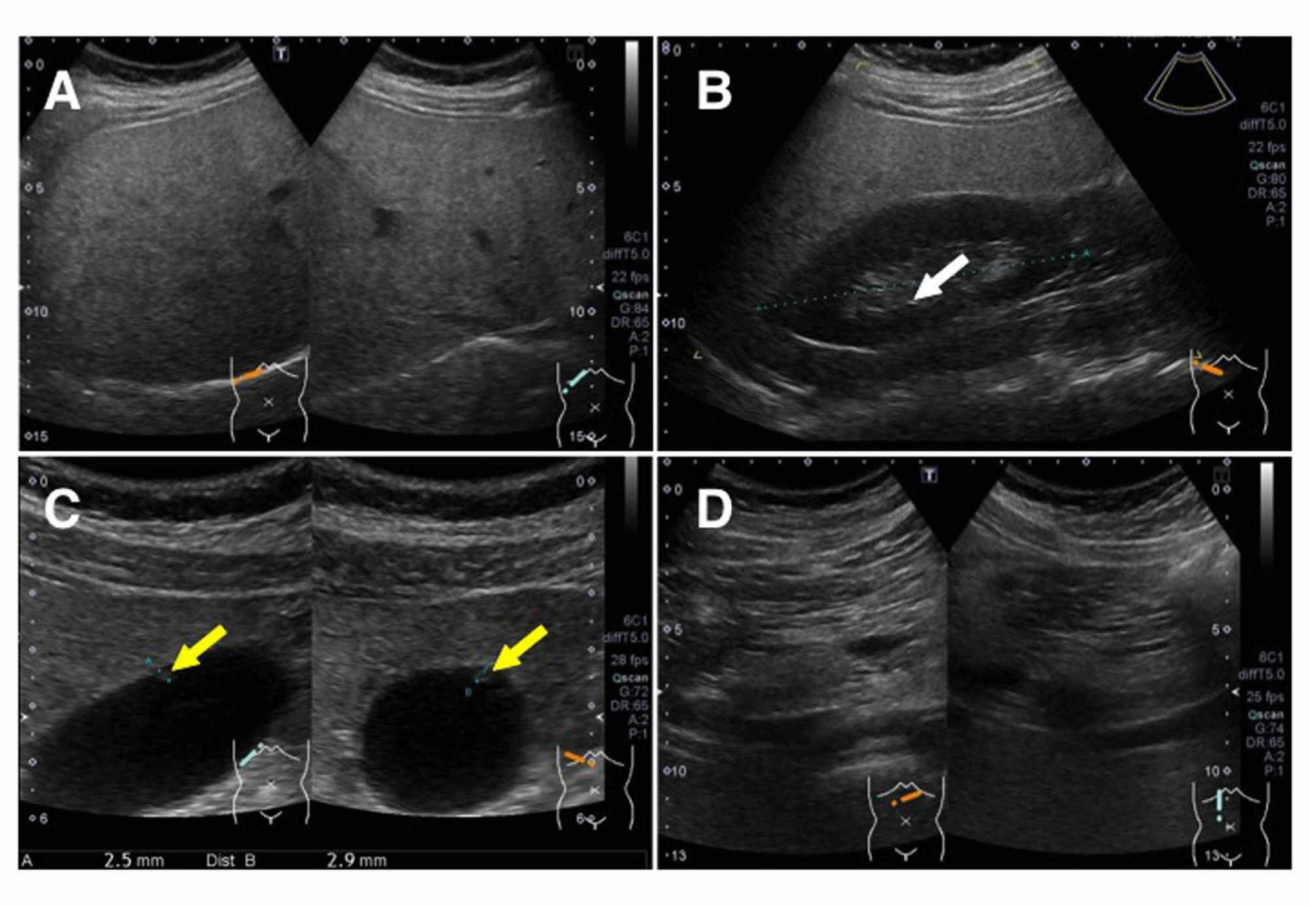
Abdominal ultrasound images before therapy A: Liver images; B: Liver and right kidney image, white arrow indicates kidney calcification; C: gallbladder image, yellow arrows indicated a gallbladder polyp; D: pancreas images
